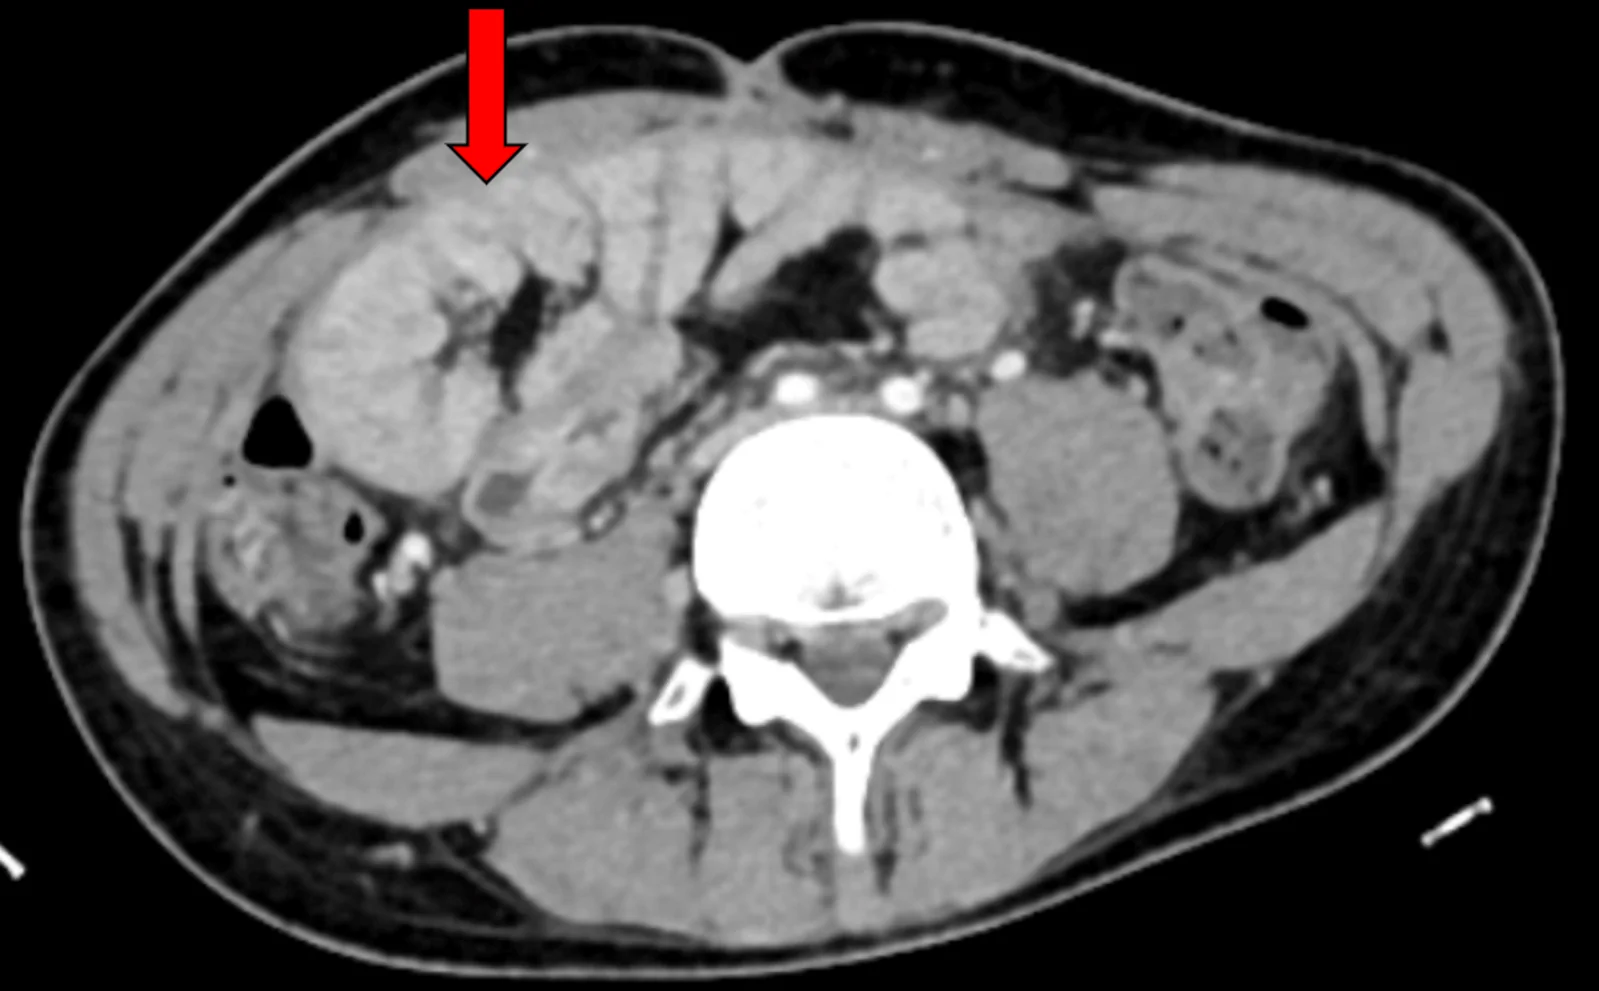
Axial CECT image of the patient demonstrates centrally clustered small bowel loops enclosed by a smooth, enhancing fibro-collagenous membrane (red arrow), characteristic of EP (abdominal cocoon syndrome) CECT: contrast-enhanced computed tomography; EP: encapsulating peritonitis
